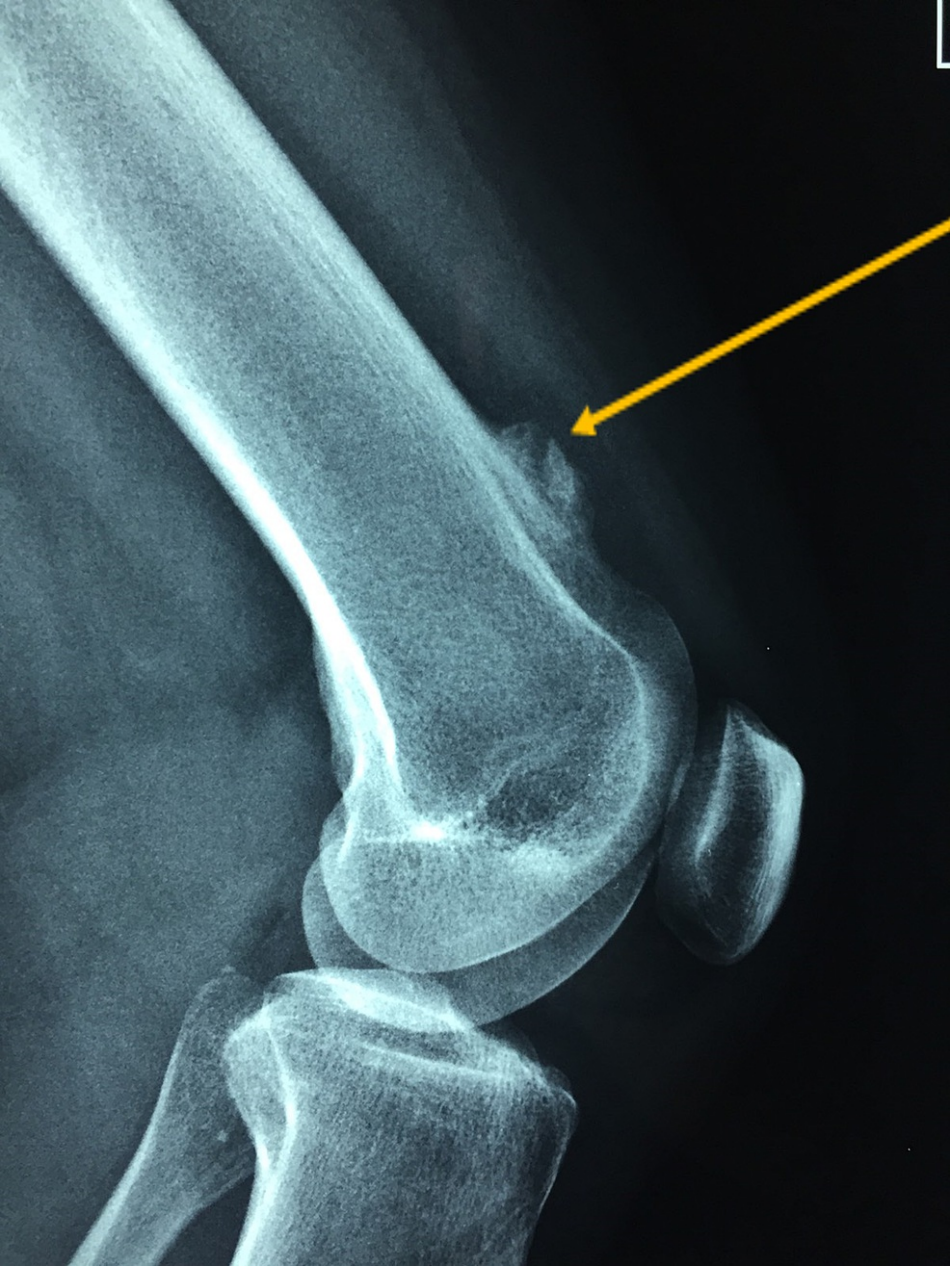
Lateral radiograph of the patient's knee where the bony mass can be seen (yellow arrow).
